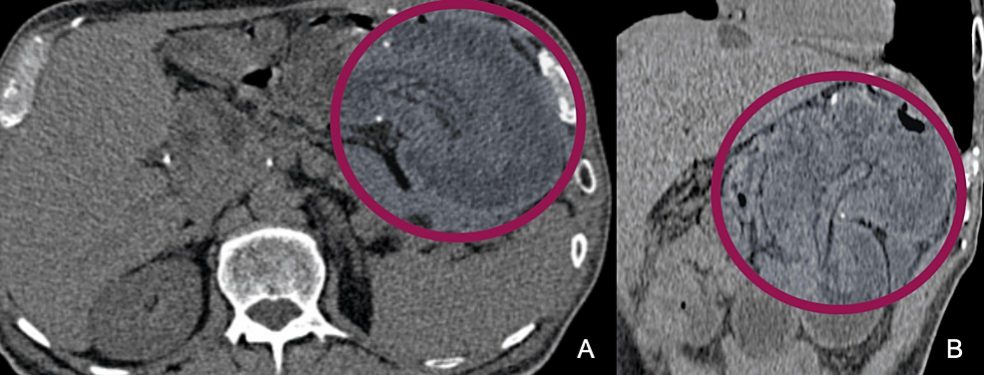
Abdominal CT. Contrasted abdominal axial CT showing intestinal intussusception at the level of the gastrojejunal anastomosis within the stomach (circle), without subdiaphragmatic free air. (A) axial section. (B) Coronal reconstruction.
